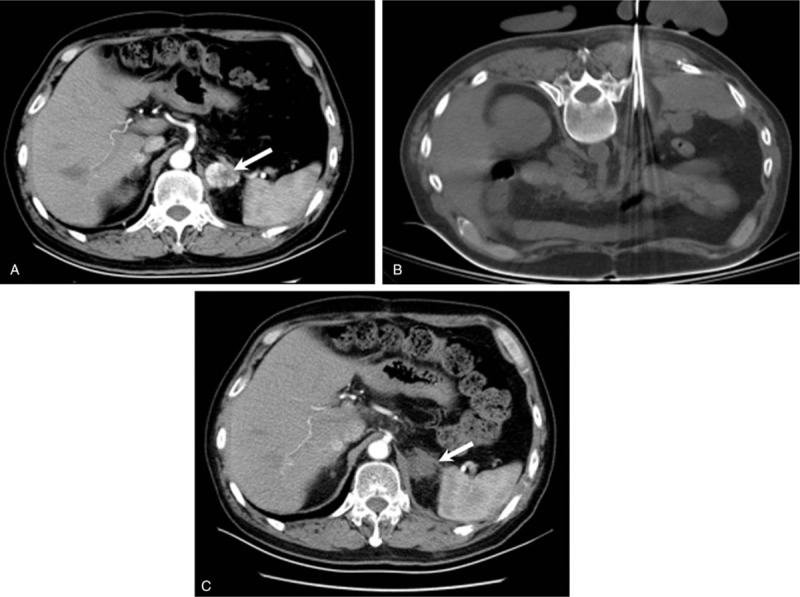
A 59-year-old man who developed left adrenal metastasis after the resection of renal clear cell carcinoma. (A) Contrast-enhanced CT scan showing a tumor (arrow) in the left adrenal gland. (B) Procedures for cryoablation. (C) Contrast-enhanced CT scan showing that the tumor enhancement is no longer visible (arrow). CT = computed tomography.
